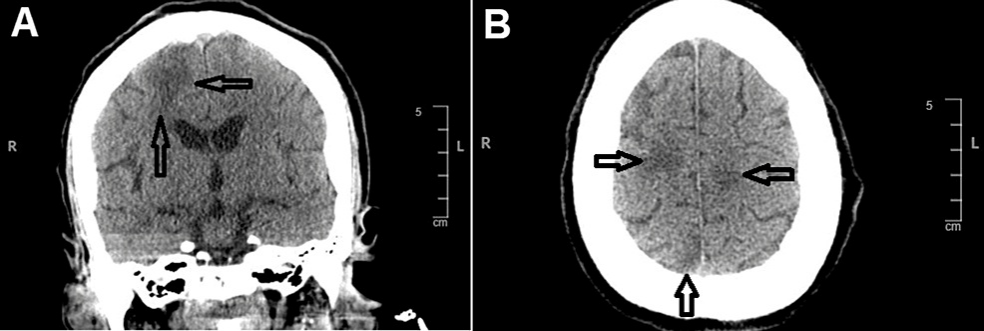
CT of the head without contrast displaying hypodensity in the right parietal cerebral cortex in coronal view (A) and the cerebral hemispheres in axial view (B).
